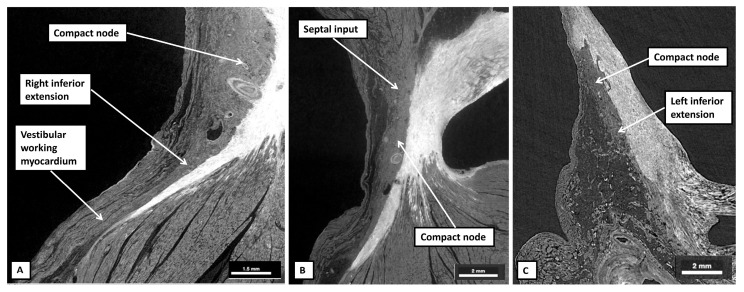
The images are taken from a human heart scanned using HiP-CT at the European Synchrotron Radiation Facility and made available at 6.51 um from the heart of the body donor S20-29. (A) Panel A shows the transition between the compact node and the rightward extension into the right atrial vestibule, together with the merge-point between the rightward extension and working myocardium. (B) Panel B shows the input to the compact node from the atrial septum, in other words the fast-pathway, with minor infiltration by fat separating it from the right atrial vestibule. (C) Panel C shows the much smaller leftward extension from the compact node and the merge-point with the working myocardium of the left atrial vestibule.
